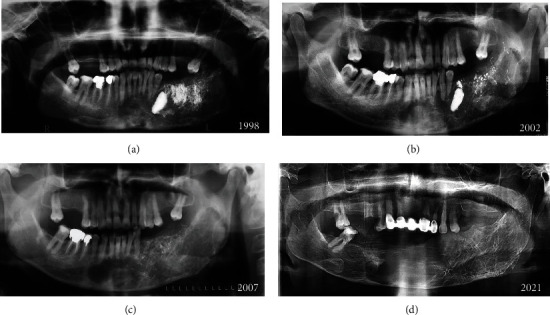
(a) The same day panoramic radiograph. (b) 4-year panoramic radiograph. (c) 9-year panoramic radiograph. (d) 22-year panoramic radiograph; note the calcification inside the lesion.
